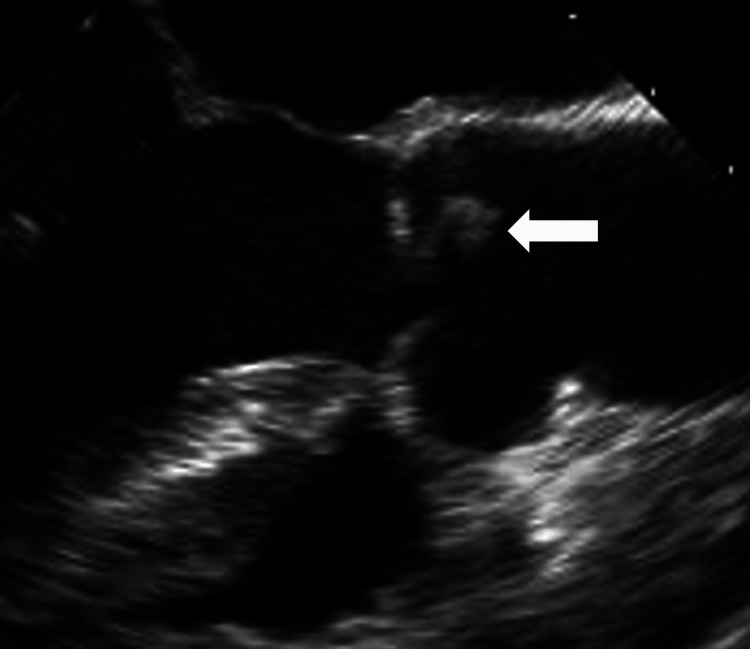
Transesophageal echocardiography image with the mid-esophageal aortic valve long axis view A pedunculated, oval-shaped, isoechoic, and mobile mass at the non-coronary cusp of the aortic valve was revealed (arrow).
